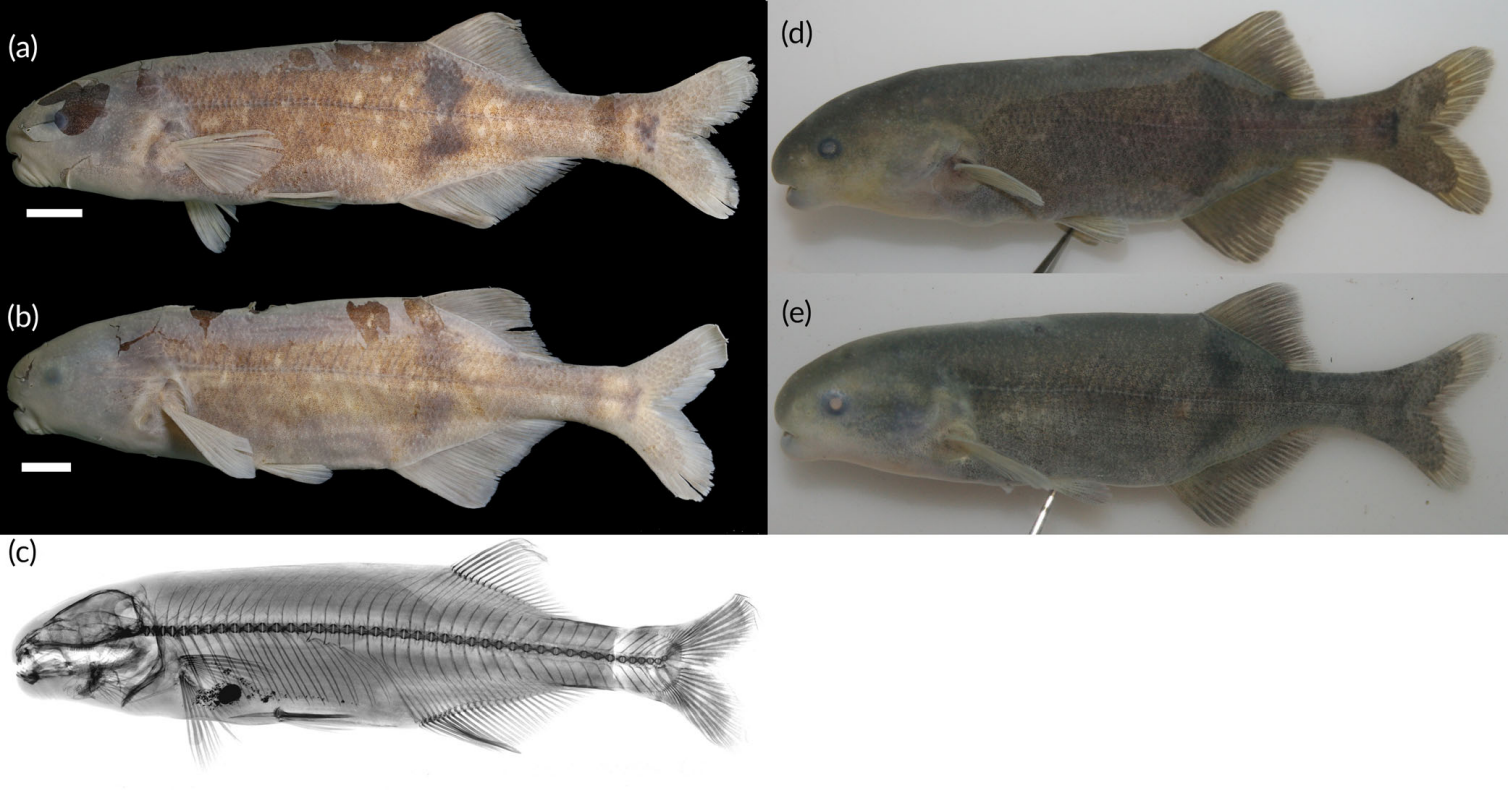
(a) *Heteromormyrus pauciradiatus* (SAIAB 85209), female 118.7 mm standard length (SL), from the Kwanza River; (b) *H. pauciradiatus* (SAIAB 85203), male 108.7 mm SL, from the Kwanza River; (c) X‐ray radiograph of holotype; (d, e) live pictures of *H. pauciradiatus*. Scale bar =1 cm.
